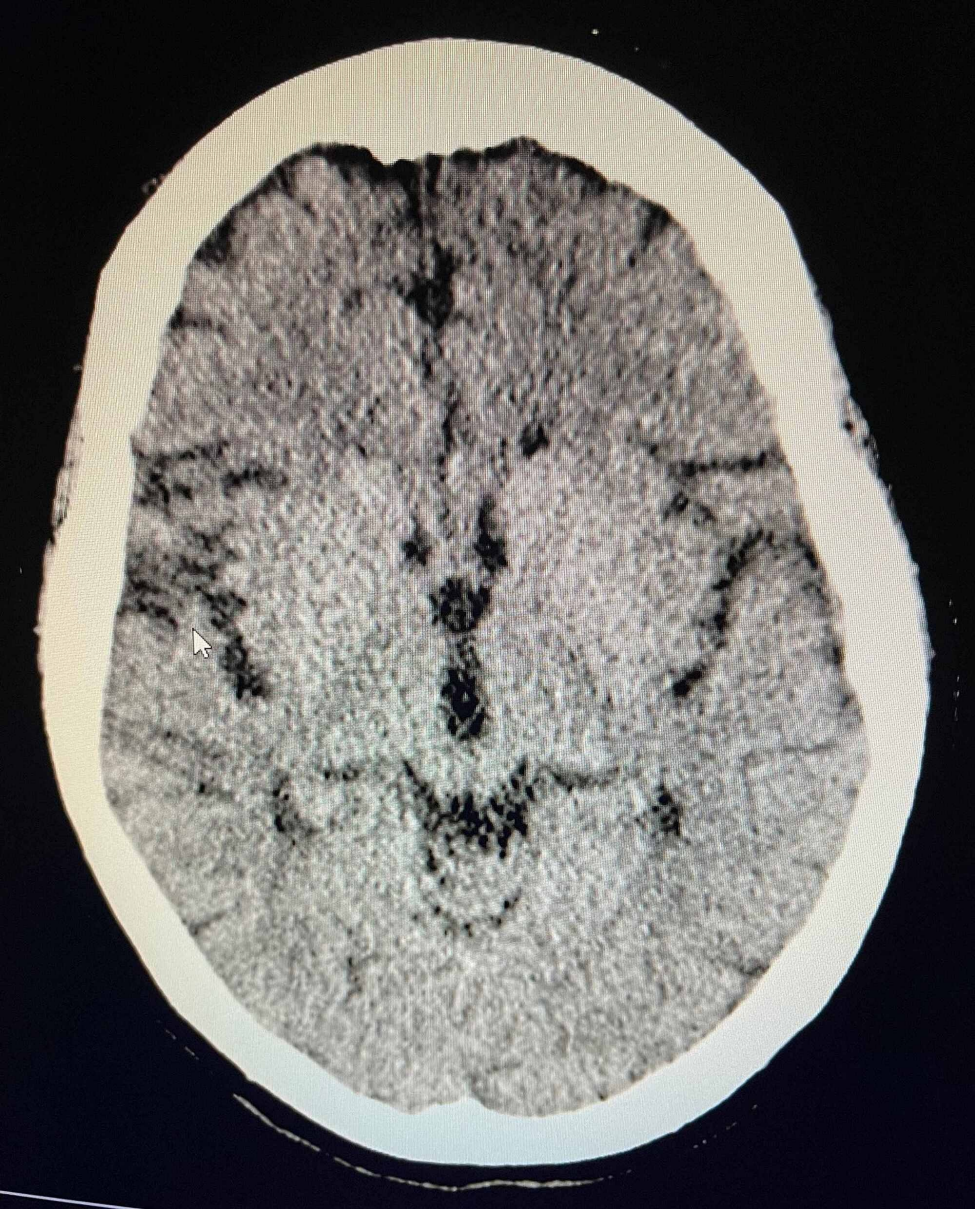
Computerized tomography (CT) scan of the head showing cortical atrophy and mild chronic small vessel white matter ischemic disease and an old infarct in the right posterior parietal lobe.
